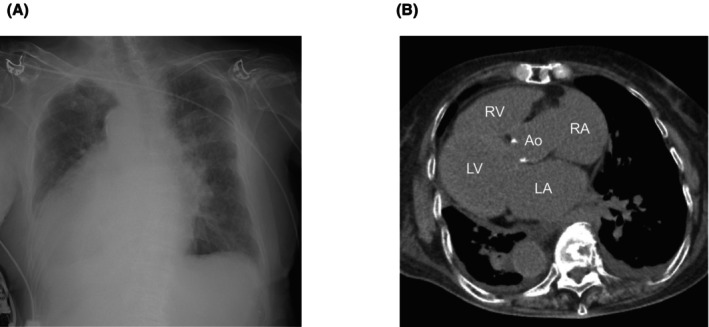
Both the chest radiograph (posteroanterior view) (A) and computed tomography images (B) confirmed that the patient had dextrocardia. Ao, aorta; LA, left atrium; LV, left ventricle; RA, right atrium; RV, right ventricle.
